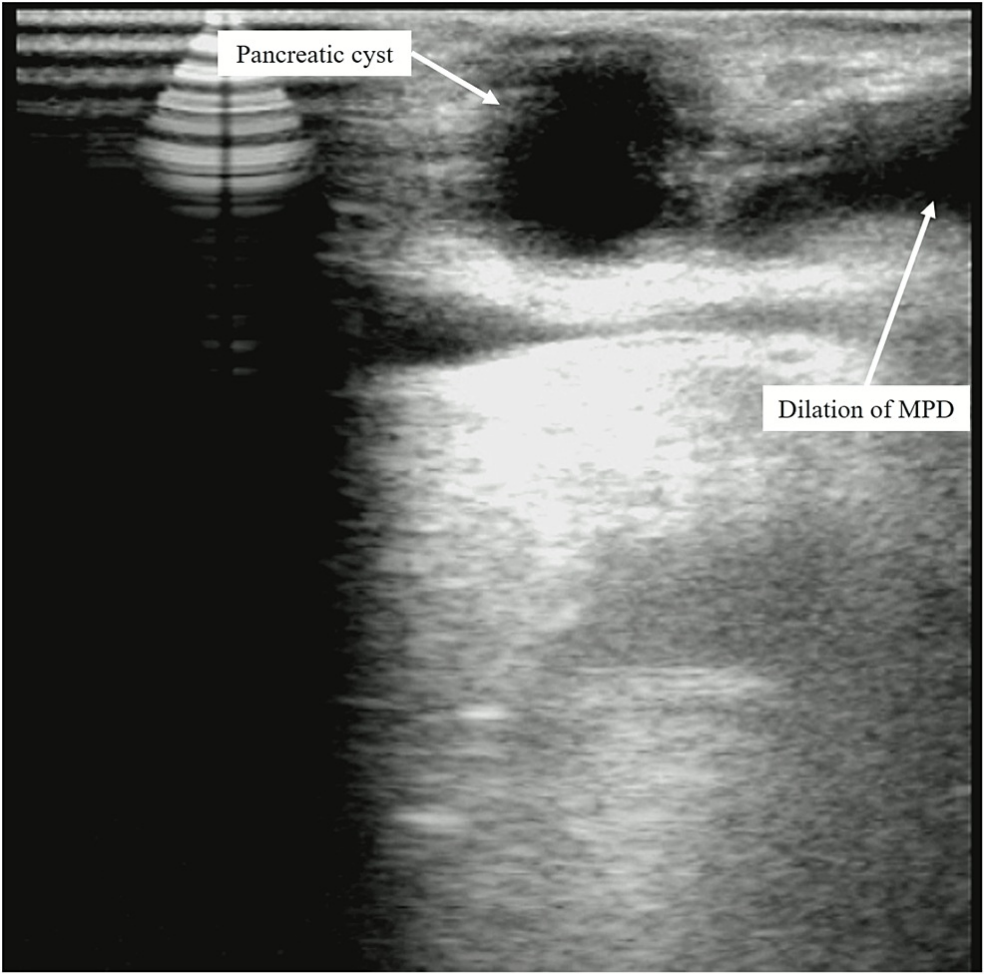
IOUS revealed a 17 mm cystic lesion in the pancreatic neck with MPD dilation. Abbreviations: IOUS, intraoperative ultrasound; MPD, main pancreatic duct.
